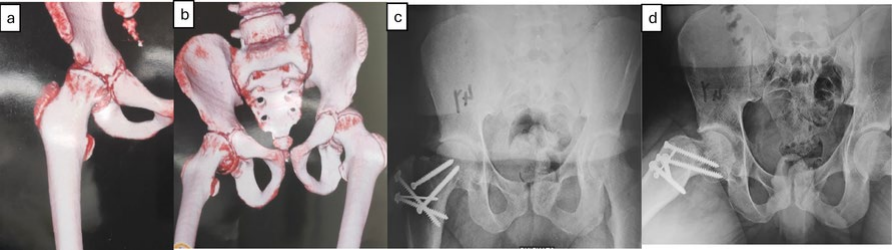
a, b Preoperative radiographs 3D CT showing right SCFE in 11-year-old boy. c, d Postoperative radiographs (ap and lateral) at final follow-up visit (7 years) and after fixation by two cannulated screws
